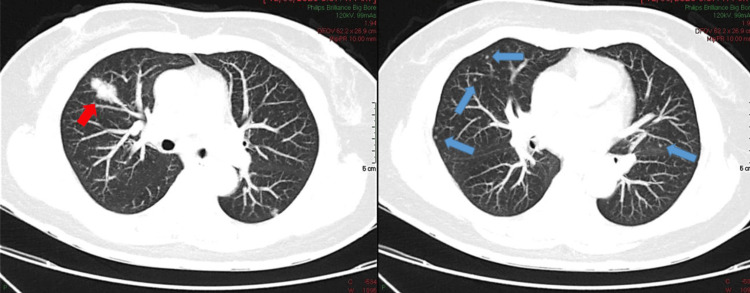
Baseline axial HRCT showing a 28 × 20 mm irregular opacity in the right upper lobe (red arrow) with multiple bilateral micronodules (blue arrows) HRCT: high‑resolution computed tomography
